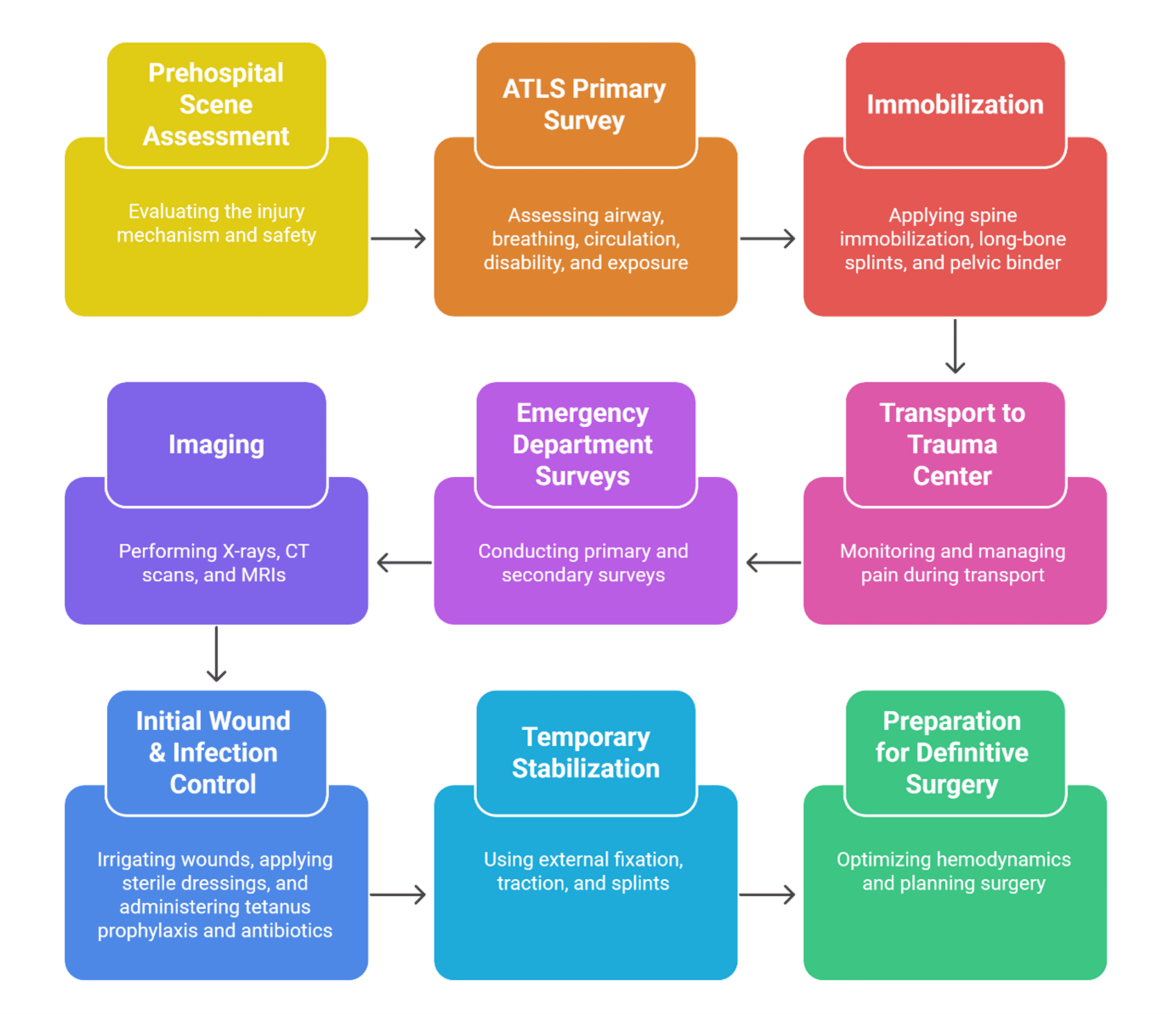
Pathway of early orthopedic trauma assessment and stabilization ATLS: advanced trauma life support, CT: computed tomography, MRI: magnetic resonance imaging Image Credit: Authors
